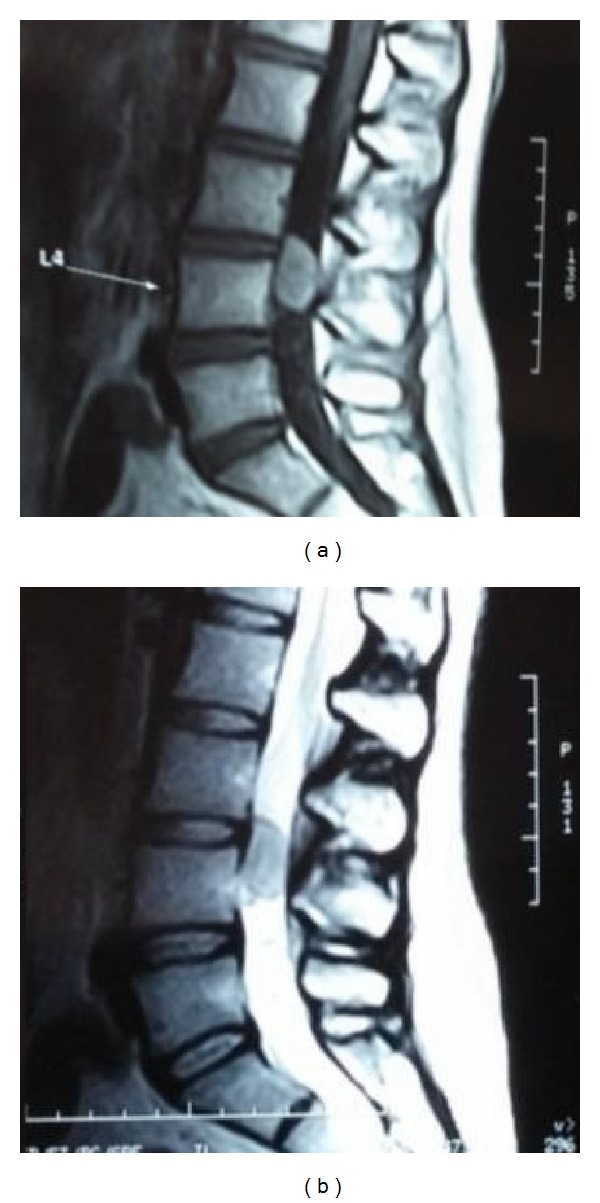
MRI of the lumbar spine showing a hyperintense (T1 weighted images (a)) and hypointense (T2 weighted images (b)) intracanalar tumor on the posterior aspect of the L3-L4 disc.
